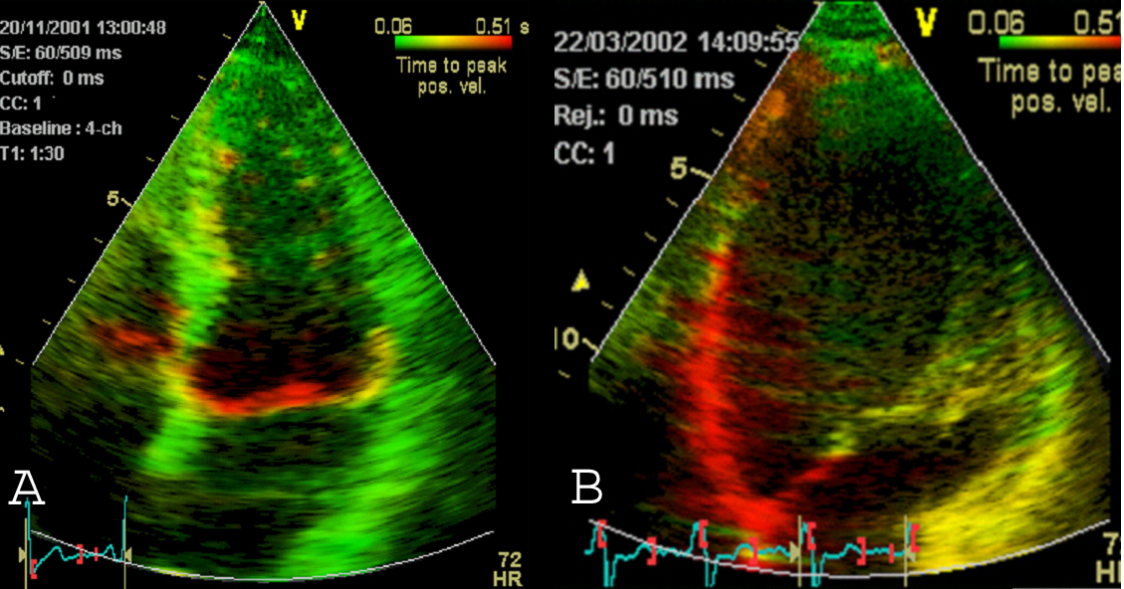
Tissue Synchronization Imaging displays colour-coded time-to-peak tissue Doppler velocities. The colour-coding is green (normal time-to-peak velocity: 20–150 ms), yellow (150–300 ms) and red (300–500 ms) Apical four chamber view. A) TSI in a control patient (only green colour coding indicating synchronous contraction) B) TSI in a patient with LBBB: The basal and mid-septal segments show a delayed time-to-peak velocity (red colour).
